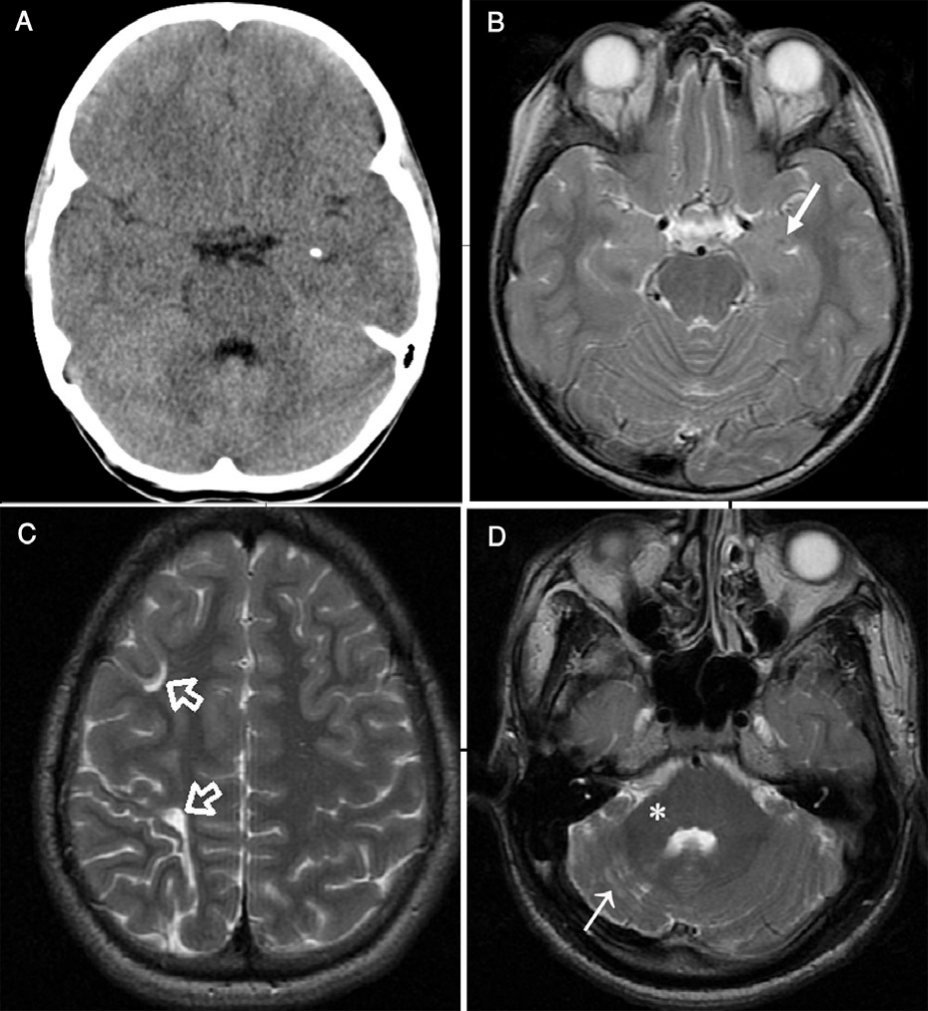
Brain CT and MRI of Patients 1 and 2. (a) Brain CT image of Patient II-6 of Family 1 at age of 10 years showing a small calcification in the left amygdale. Calcification in the right amygdale was seen on another section (not shown). (b) Brain axial T2-weighted MR image of the same patientat the level of temporal lobes showing a tiny hypointensity (arrow) representing amygdala calcification. The mesial temporal lobes were otherwise normal. (c) Brain axial T2-weighted MR images of Patient II-1 of Family 1 showing enlarged sulci and small gyri in the watershed zones of the right frontal lobe (open arrows) associated with loss of white matter due to old ischemia. (d) The right cerebellar hemisphere of the same patientwas small with widened folia, white matter gliosis (arrow), and a small right middle cerebellar peduncle (asterisk). Remodeling of the posterior fossa confirmed the long-standing nature of this abnormality. CT and MRI scans on patients from Families 2 and 3 were normal.
